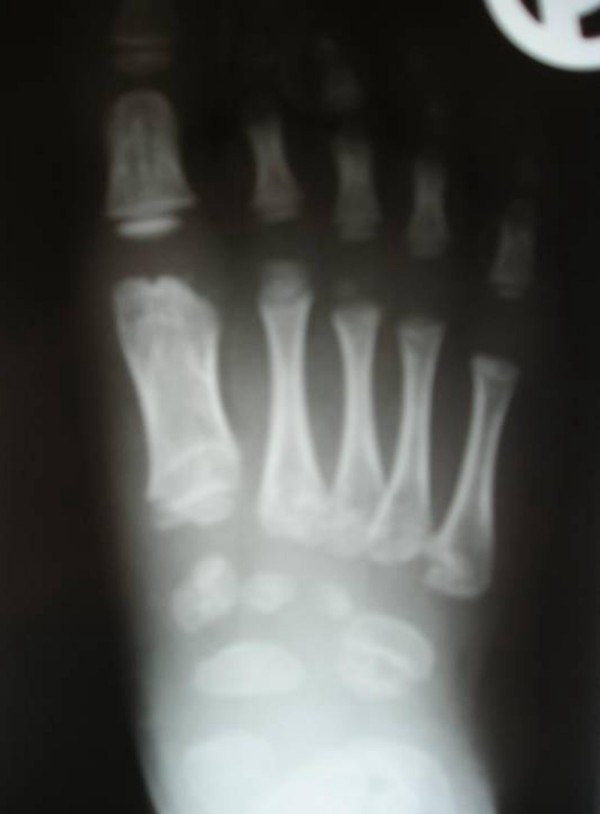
Anteroposterior foot radiograph showed defective ossification of the tarsal bones as well as brachymetatarsia and Brachyphalangia were present. There was epiphyseal clefting of the distal first and the proximal 5th metatarsophalangeal joints.
